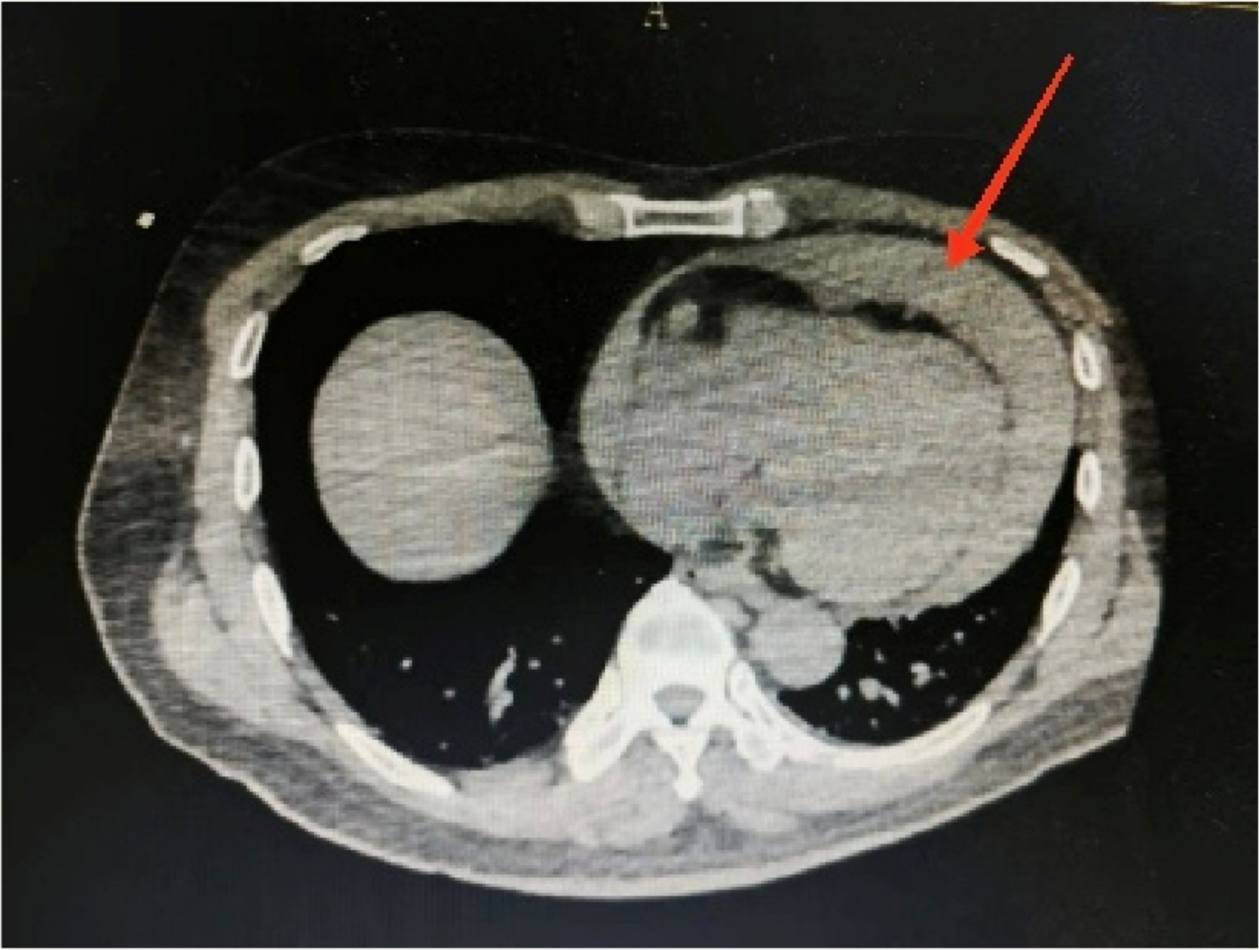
Aortic computed tomography angiography showing a massive hemorrhage of the pericardium.
